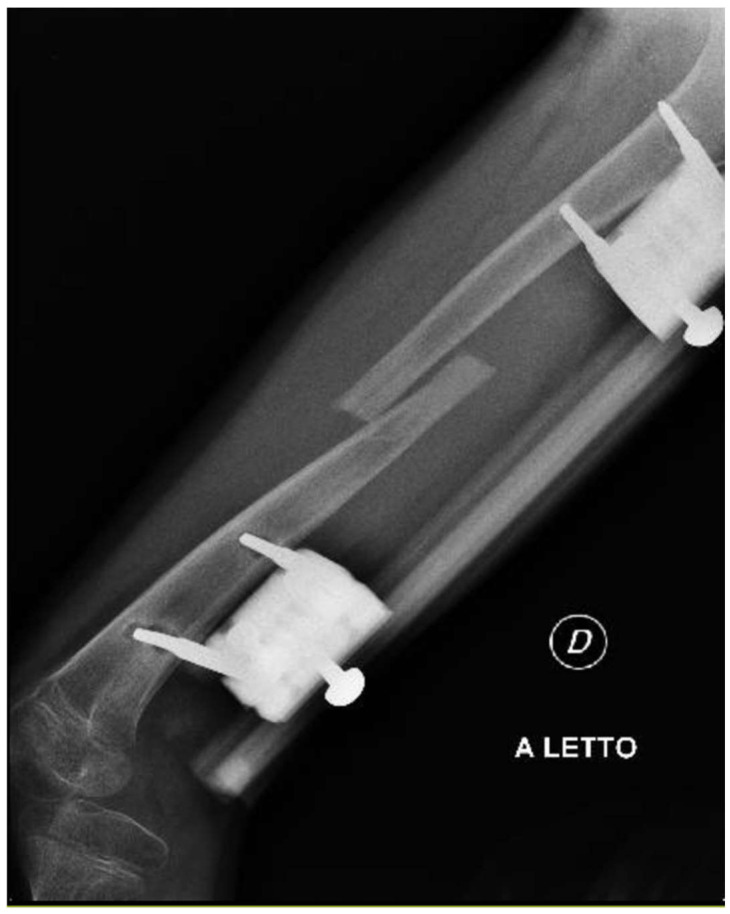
Radiographs showing fracture of the femoral regenerate.
